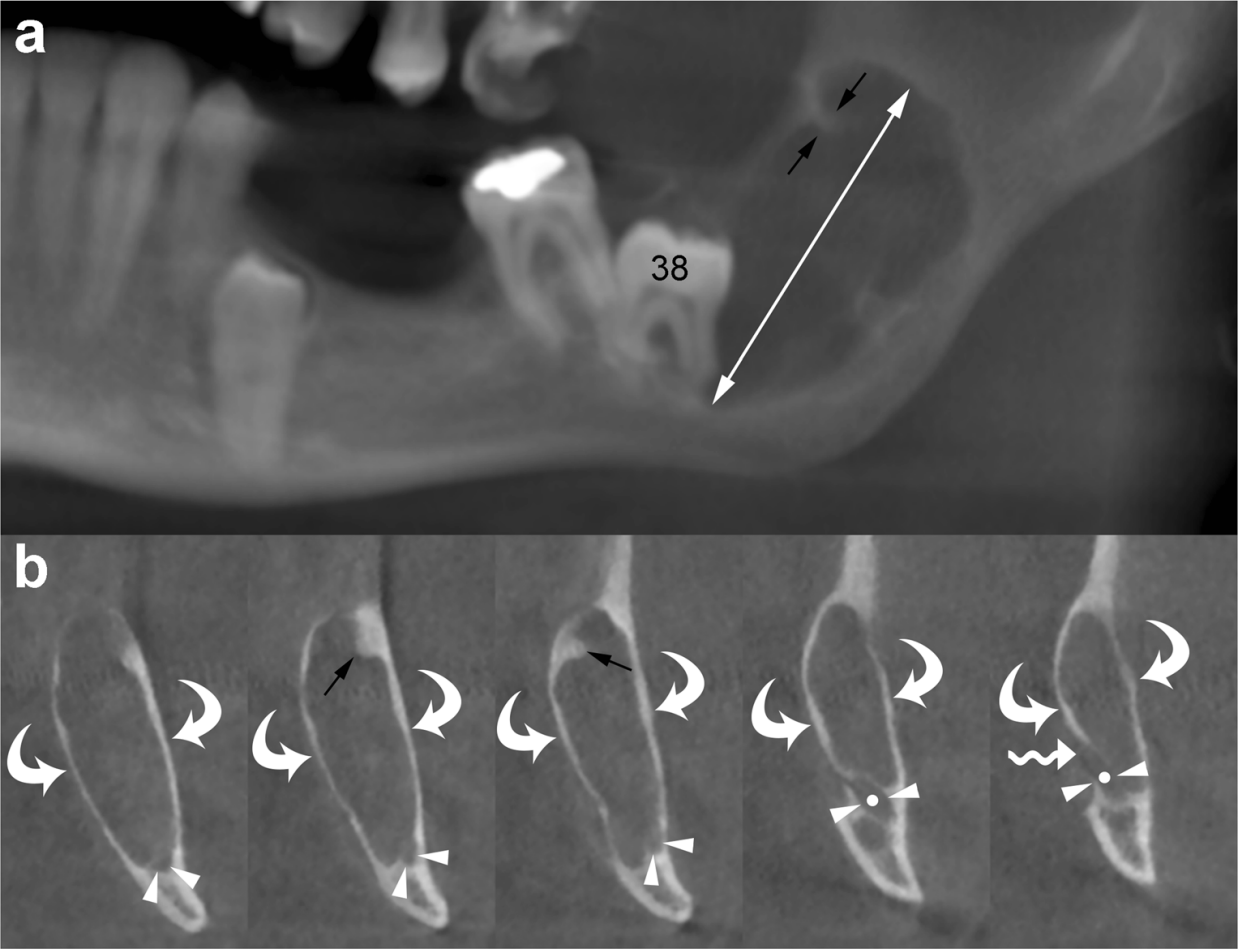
Mandibular OKC. Panoramic (a) and cross-sectional (b) cone beam computed tomography (CBCT) images display an osteolytic odontogenic lesion in the posterior left mandible and ramus, with a growth predominantly along the length of the bone (*double-headed arrow*) and minimal expansion of the buccal and lingual cortices (*curved arrows*). Note mesial displacement of the impacted third molar (*38*) and inferior displacement of the mandibular canal (*arrowheads and dots*). Small and incomplete internal septum (*small black arrows*) due to the endosteal scalloping of the cortical plate are also shown. *Wavy arrow*, mandibular foramen
